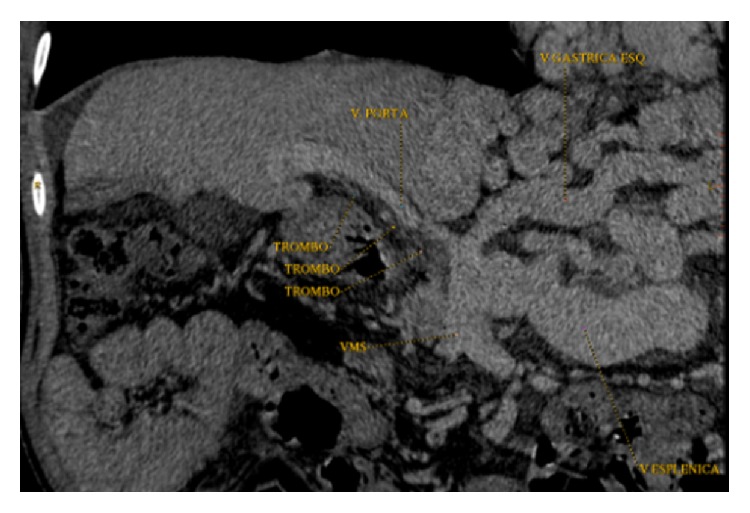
Preoperative computed tomography showing extensive thrombosis of portal and superior mesenteric veins and enlarged left gastric vein draining the bowel.
